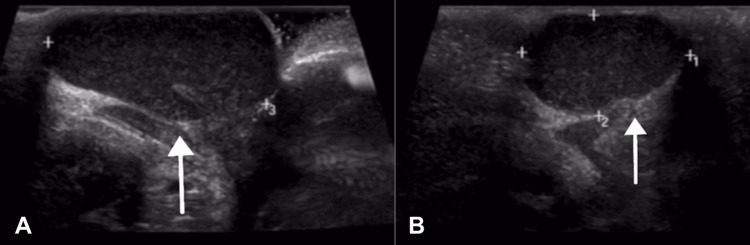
Ultrasonography of the clitoral swelling showing a hypoechoic lesion measuring 2.6 x 1.6 x 3.5 cm (A, B).
